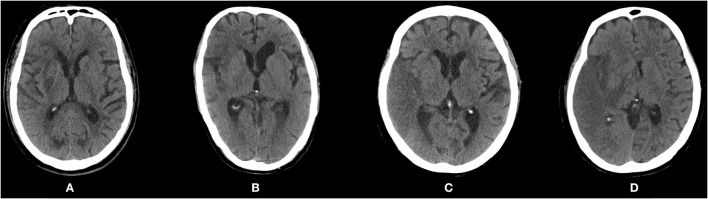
Post-procedure computed tomography scans showing different severity of brain edema according to the Safe Implementation of Thrombolysis in Stroke-Monitoring Study (SITS-MOST). (A) CED-0: no edema. (B) CED-1: focal brain edema up to one-third of the hemisphere. (C) CED-2: brain edema greater than one-third of the hemisphere. (D) CED-3: brain edema with midline shift. CED, cerebral edema.
